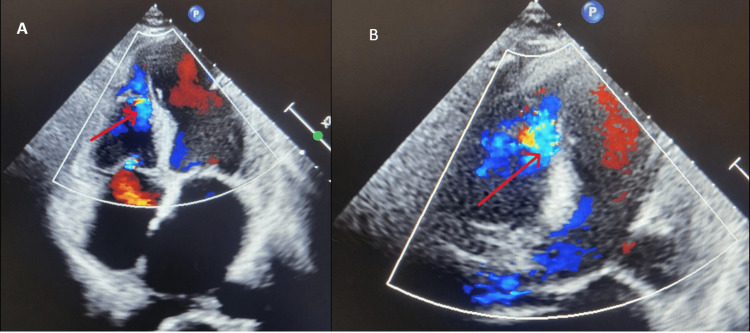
(A) Apical four-chamber view of the echocardiographic image showing a jet across the distal interventricular septum (ventricular septal rupture). (B) Akinetic distal interventricular septum and lateral wall with the ventricular septal rupture.
